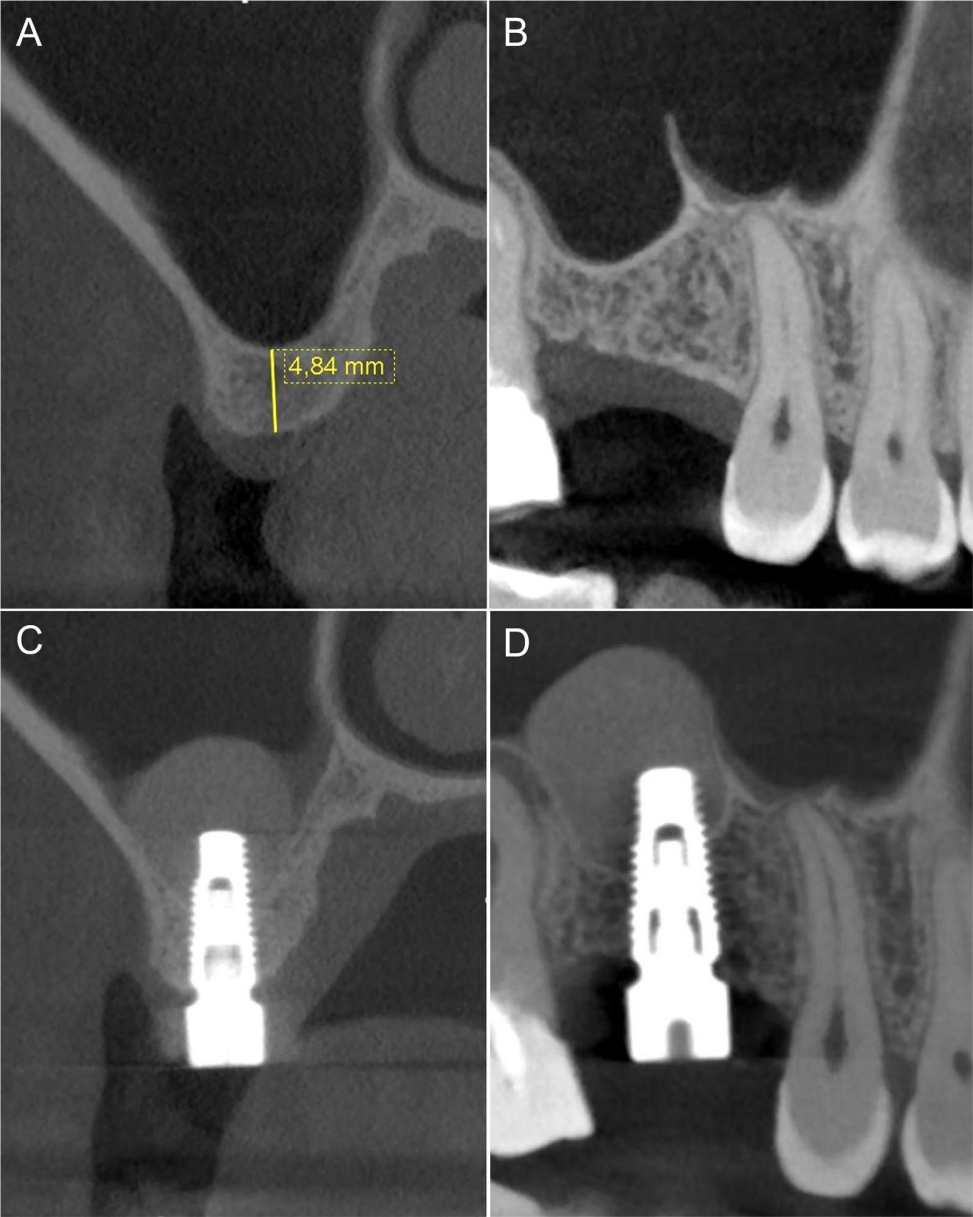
Pre-surgical CBCT with cross-section showing a narrow sinus anatomy and RBH < 5 mm (A) and panorex image highlighting the presence of a sharp Underwood septum (B). Post-operative CBCT with cross-section showing abundant amount of gel graft apically to implant apex to counteract the expected shrinkage during the healing phase (C) and panorex confirming sinus membrane integrity (D)
